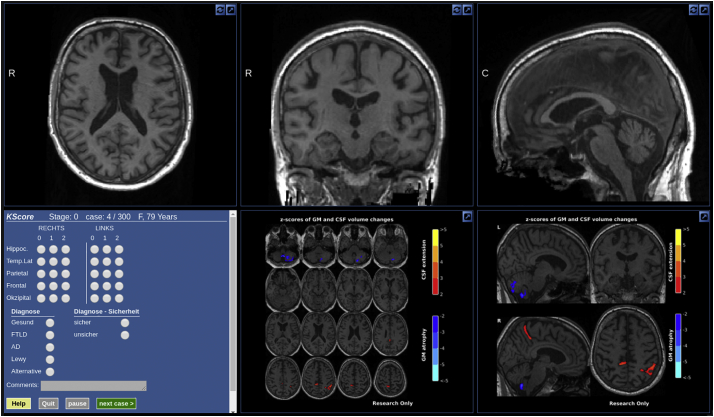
Web interface for readers showing T1 image, atrophy scores, rating questionnaire, and basic demographic information. The interface allowed to scroll through the slices of the structural MR image in all orthogonal views, to adjust the contrast and to increase each subpanel to full-screen. The sections showing the atrophy scores (bottom right on this panel) were static but could also be enlarged to fill the screen.
